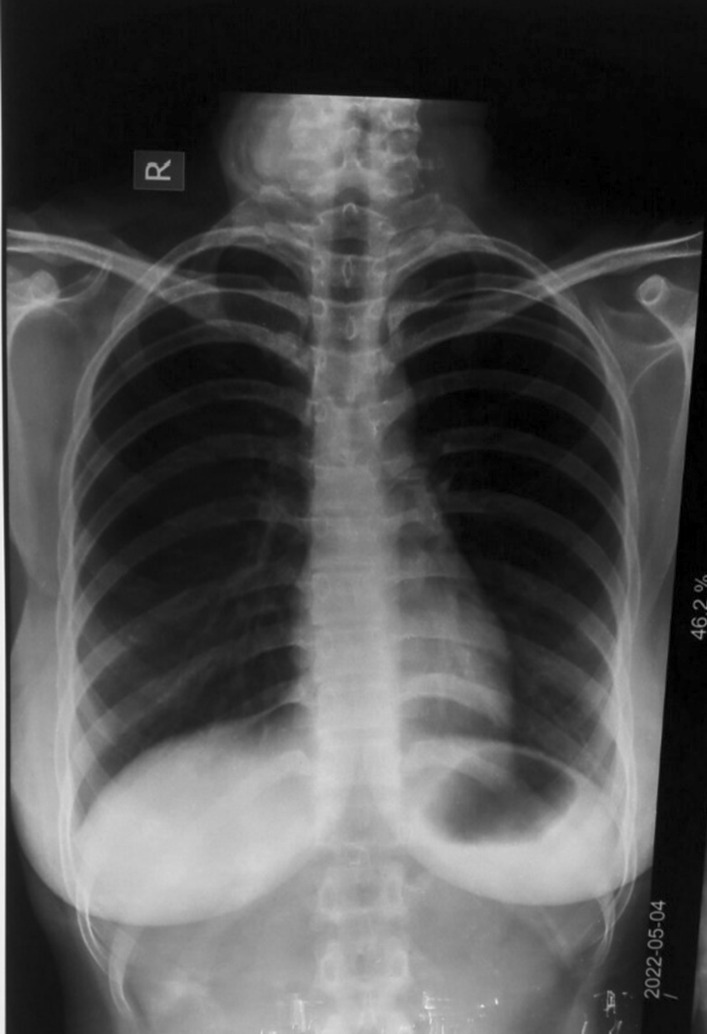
Chest x-ray showing lower lobe infiltrates
